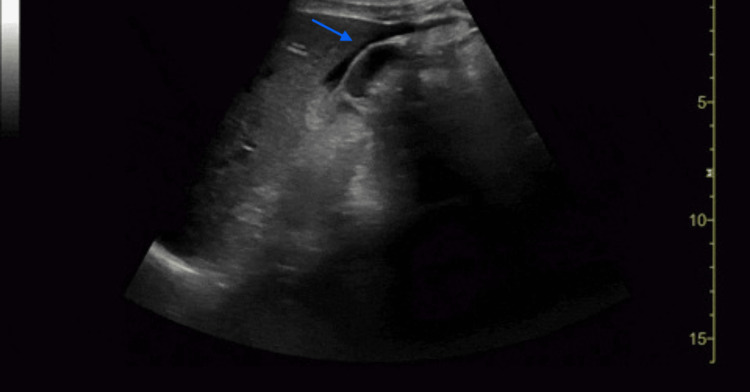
RUQ abdominal ultrasound with free fluid Anechoic fluid is identified by the blue arrow near the edge of the liver. RUQ, right upper quadrant
